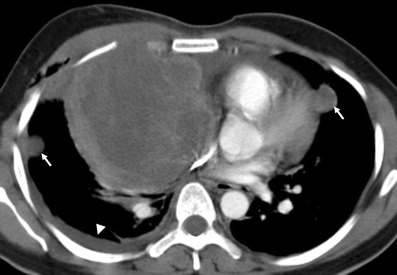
A 19-year-old man with seminoma. Contrast-enhanced axial CT scan demonstrates a large mass in the right side of the mediastinum with an obvious mass effect on great vessels and heart. The mass shows heterogeneous CT-attenuation values probably secondary to haemorrhage and coagulation necrosis. Note also a right pleural effusion (*arrowhead*) and multiple lung metastasis (*arrows*)
